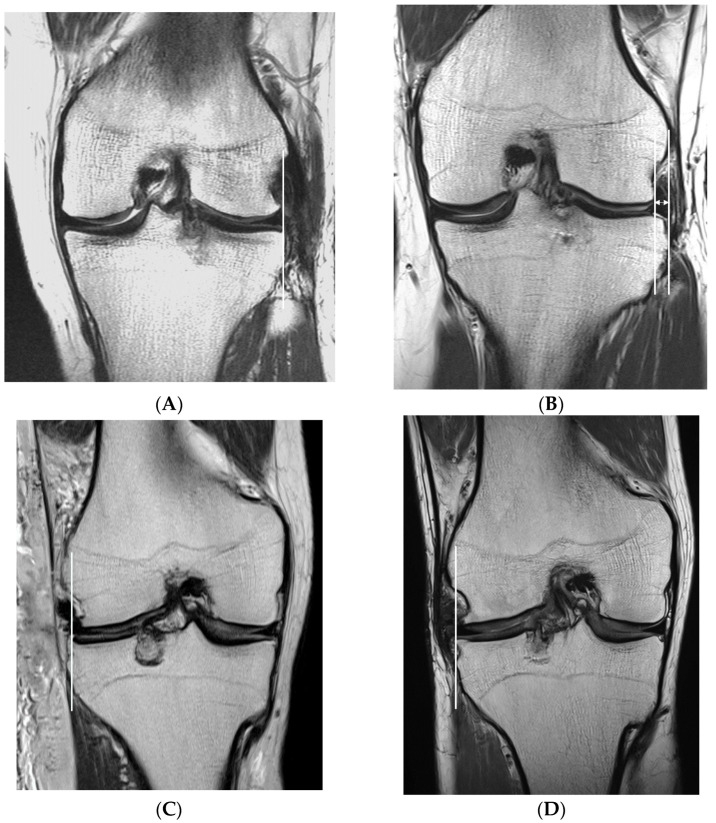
(A) Postoperative magnetic resonance image (MRI) at 2 days after lateral meniscal allograft transplantation (MAT) and (B) postoperative MRI at 1 year after the surgery in the patient who performed isolated lateral MAT. Graft extrusion increased from 0mm to 2.9mm. (C) Postoperative MRI at 2 days after lateral MAT and (D) postoperative MRI at 1 year after the surgery in the patient who performed lateral MAT + capsulodesis. Graft extrusion was not found until 1 year after the surgery.
